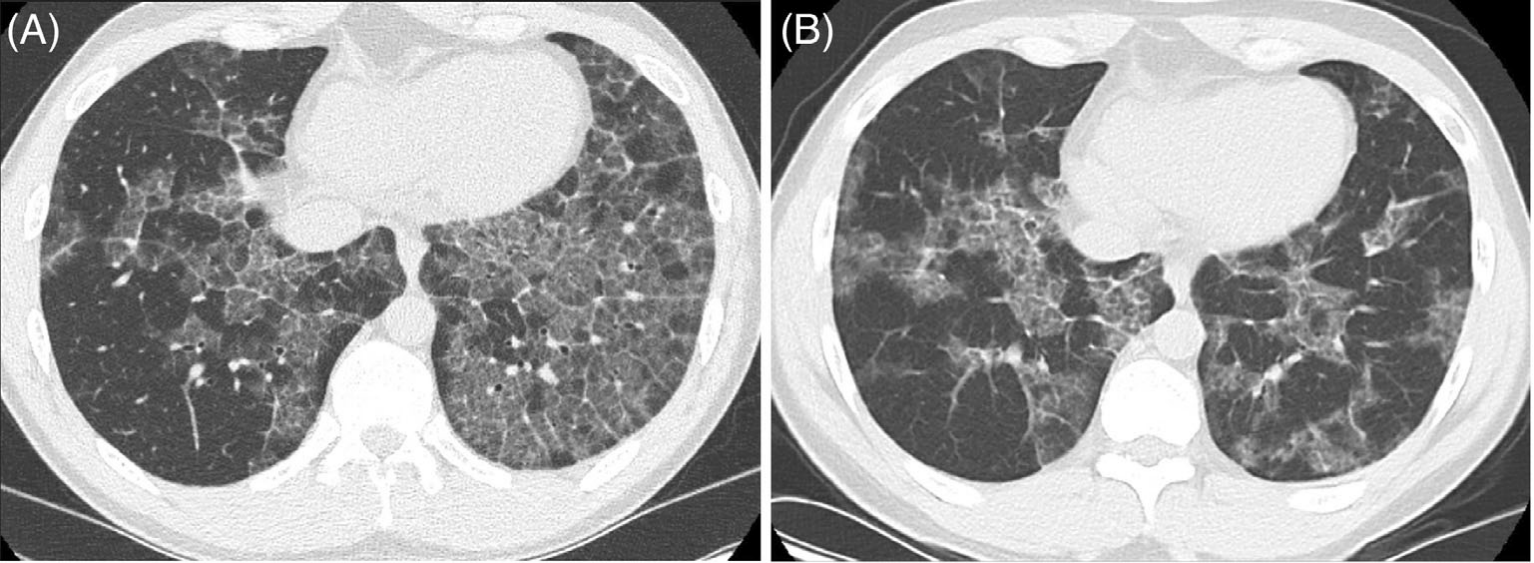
(A) Initial CT chest at diagnosis. (B) CT chest 2 years after eliminating silica dust exposure
